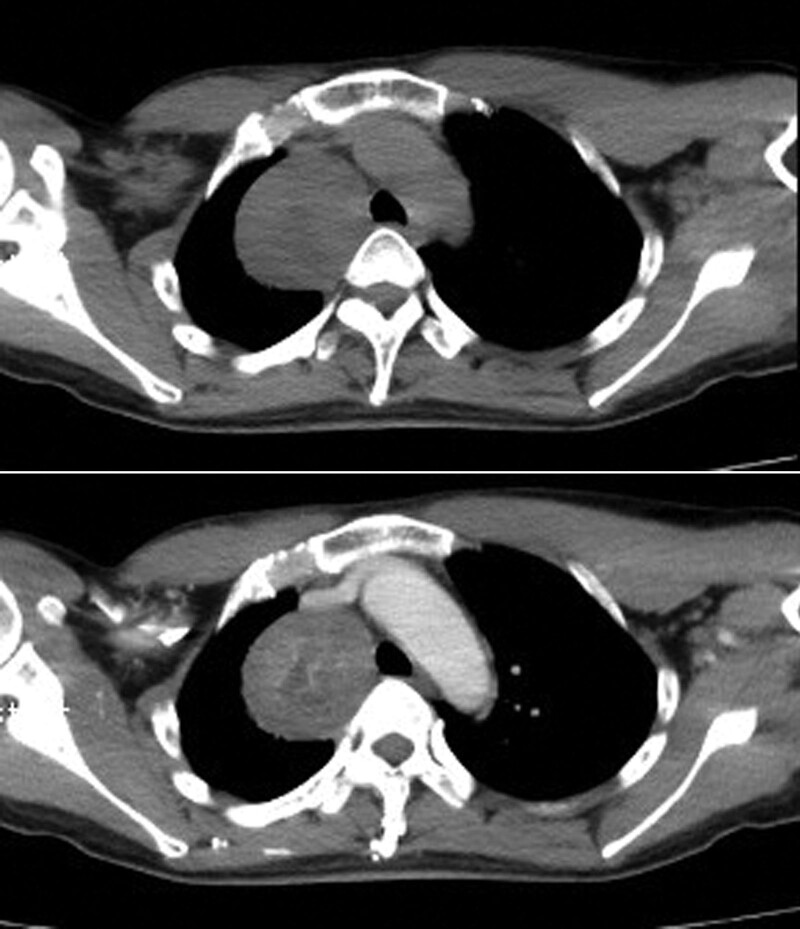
Chest computed tomography revealing a well-circumscribed, homogeneous, soft tissue mass lesion in the right upper posterior mediastinum, contrast CT showed mildly heterogeneous enhancement.
